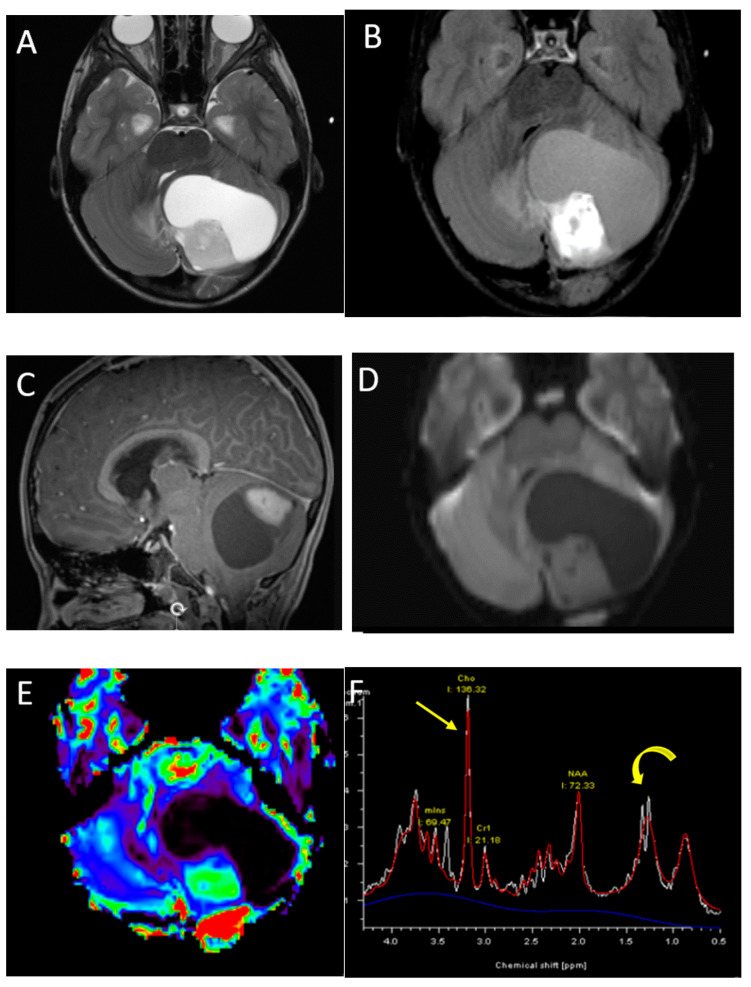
A 6-year-old boy with pilocytic astrocytoma. Axial T2 (A), post-contrast T2-FLAIR (B) sagittal post-contrast T1 (C) and axial DWI (D) images demonstrate a characteristic large cystic lesion in the left cerebellar hemisphere with a solid nodule. The nodule enhances intensely without significant diffusion restriction compared with adjacent parenchyma. Perfusion imaging (E) demonstrates significantly increased perfusion of the solid nodule. MRS (F) shows elevated choline (arrow) and high lipid lactate peaks (curved arrow).
